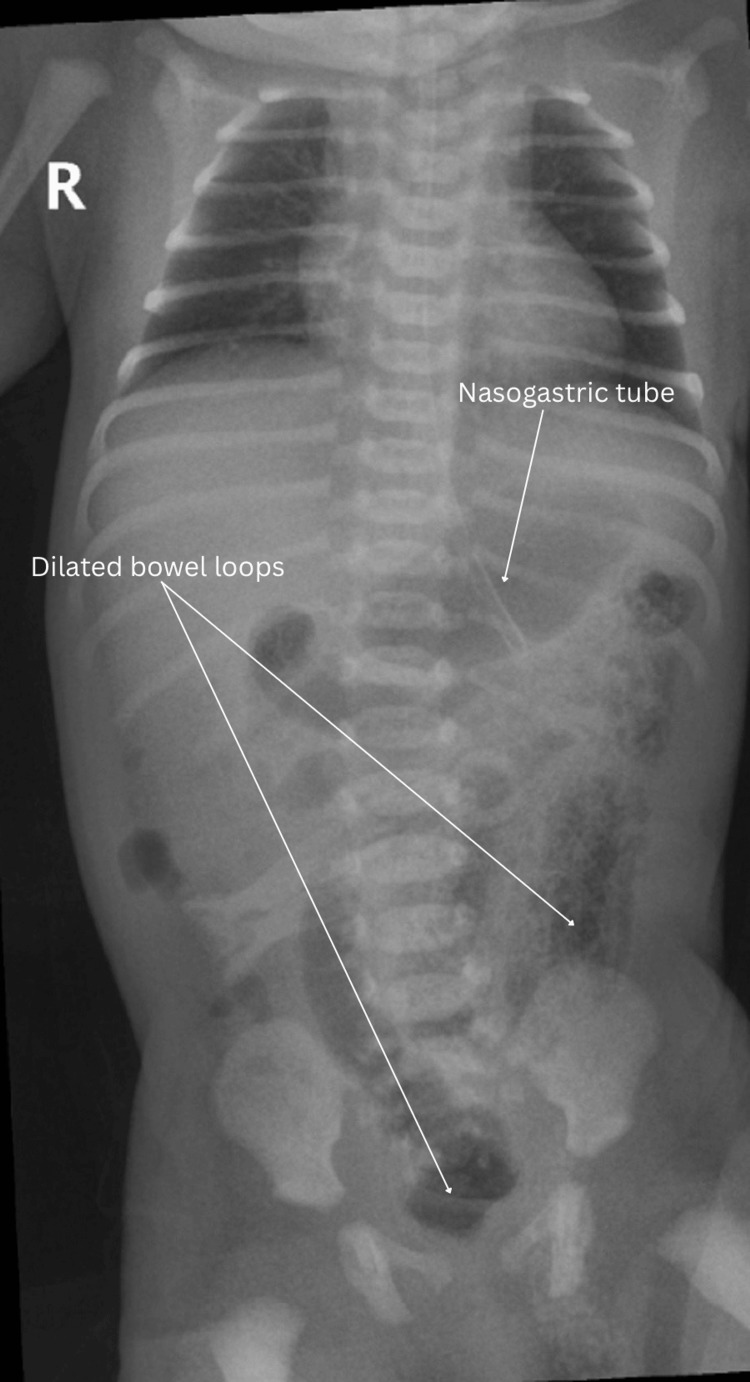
Anteroposterior chest and abdominal radiograph showing mildly dilated bowel loop, nonspecific in appearance, with preserved distal bowel gas.
